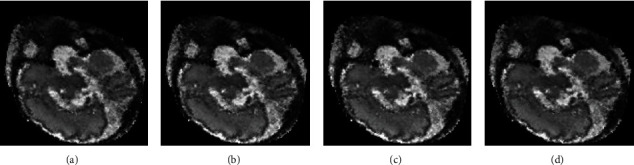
Reconstructed results of the MRI data with different window sizes *w*: (a) *w* = 3, (b) *w* = 5, (c) *w* = 7, and (d) *w* = 9.
